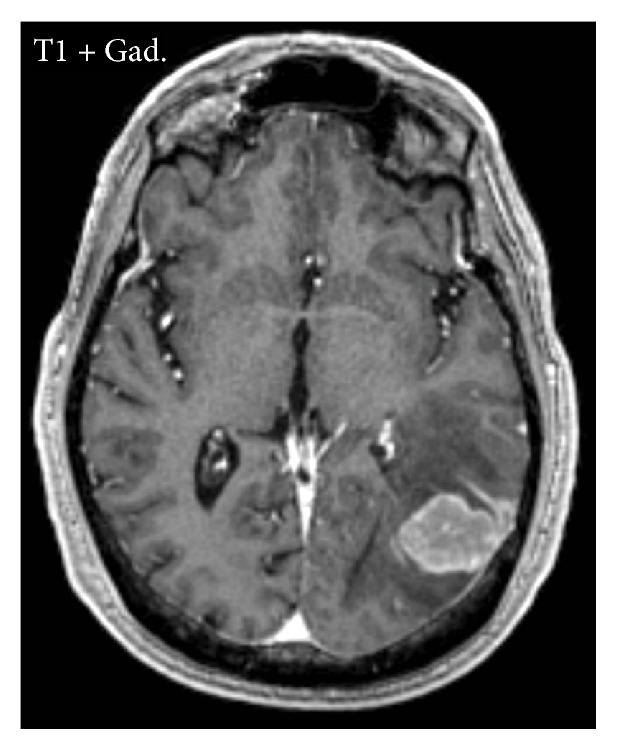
Contrast-enhanced axial T1-weighted MRI reveals a uniformly enhancing mass in the posterior temporal lobe with focal leptomeningeal enhancement.
